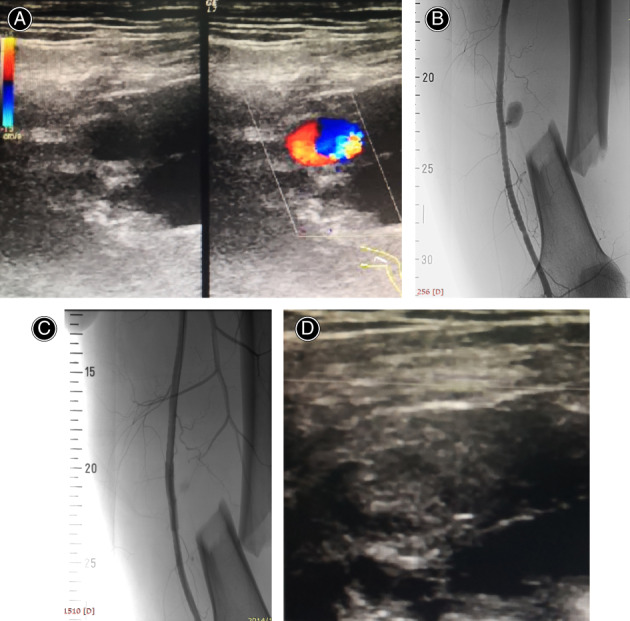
A 44‐year‐old patient with superficial femoral PA following femoral fracture at the junction of the middle and lower thirds. (A) Arterial Duplex sonography demonstrates a superficial femoral PA. (B) Angiography shows superficial femoral PA with active extravasation of contrast. (C) PA is excluded by covered stent grafting and normal blood flow in the superficial femoral artery is observed. (D) Color Duplex sonography shows normal blood flow in the superficial femoral artery with no “to‐and‐fro” blood flow in the PA sac
